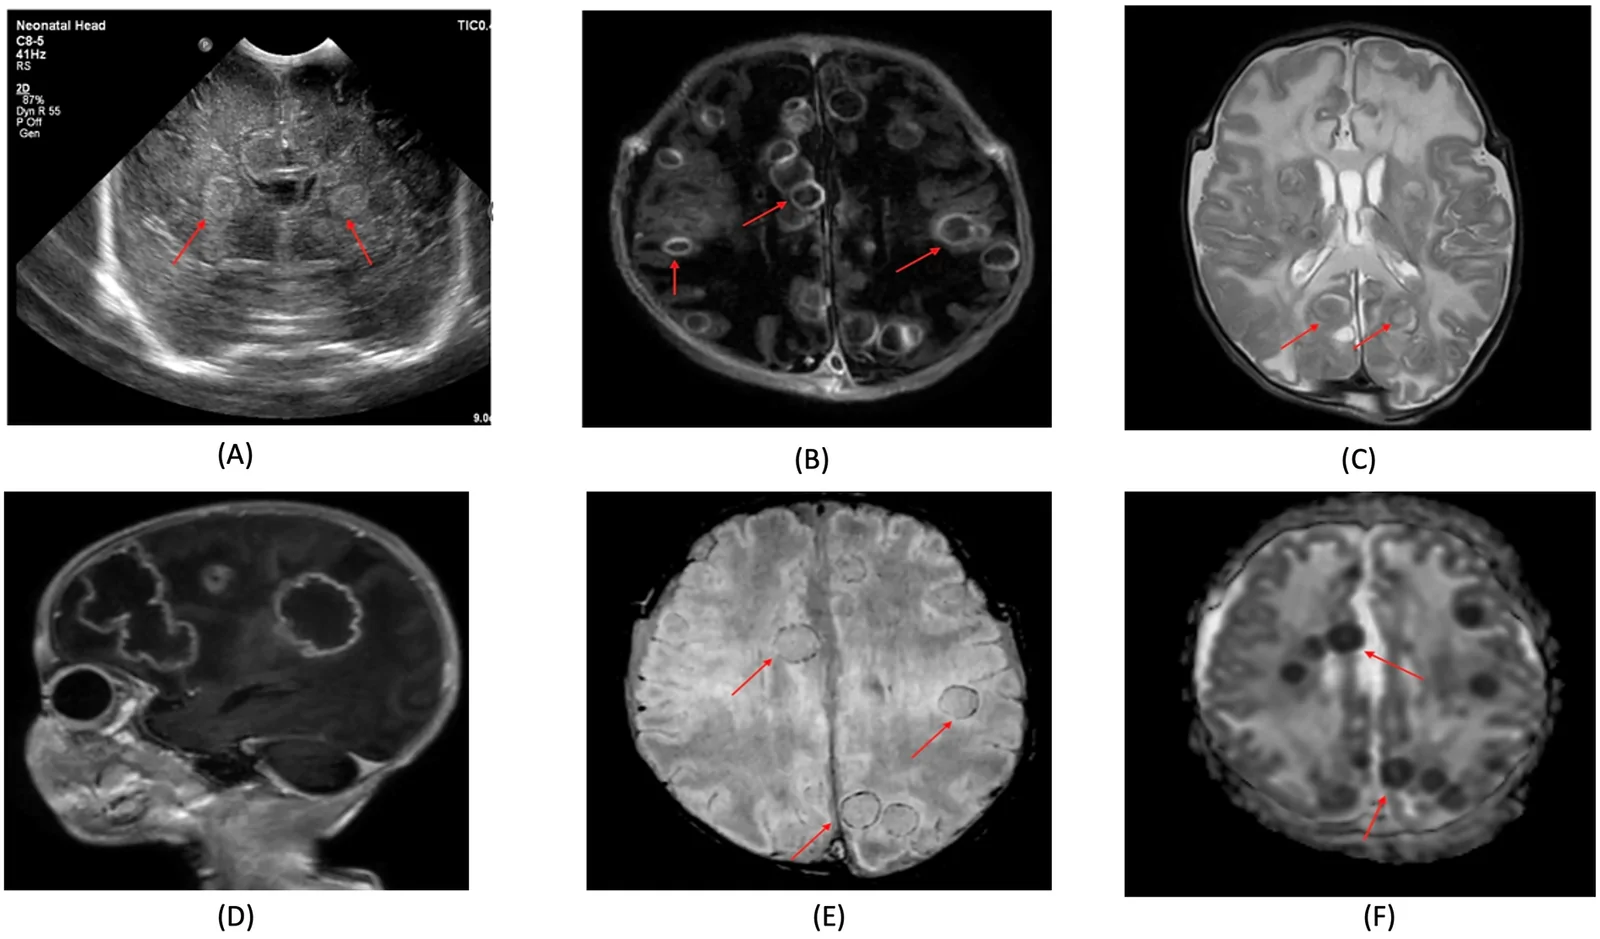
Neuroimaging findings in neonatal invasive candidiasis. (A) Cranial ultrasonography demonstrating bilateral hyperechoic lesions in the basal ganglia (arrows). (B) Axial post-contrast MRI demonstrating multiple ring-enhancing cerebral abscesses (arrows). (C) T2-weighted MRI showing focal parenchymal hyperintensities with surrounding edema (arrows). (D) Sagittal MRI depicting large cortical abscess with peripheral enhancement. (E) Susceptibility-weighted imaging demonstrating peripheral hemorrhagic areas (arrows). (F) Diffusion-weighted imaging demonstrating restricted diffusion within abscess cavities (arrows).
